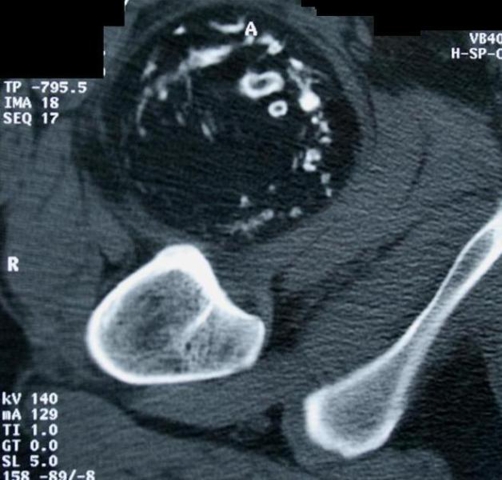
Axial CT of the right thigh showing a well-delineated, encapsulated 10 × 8 × 7 cm soft tissue mass with irregular trabeculae of bony density located in the proximal quadriceps muscle without connected to the femur.
